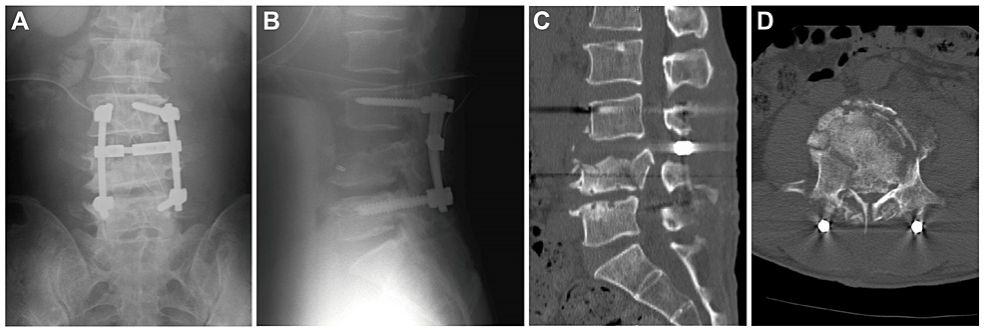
Radiographs (A: anteroposterior and B: lateral) and CT scan (C: sagittal and D: axial) after posterior fixation from L3 to L5. A bony protrusion into the spinal canal persisted.
